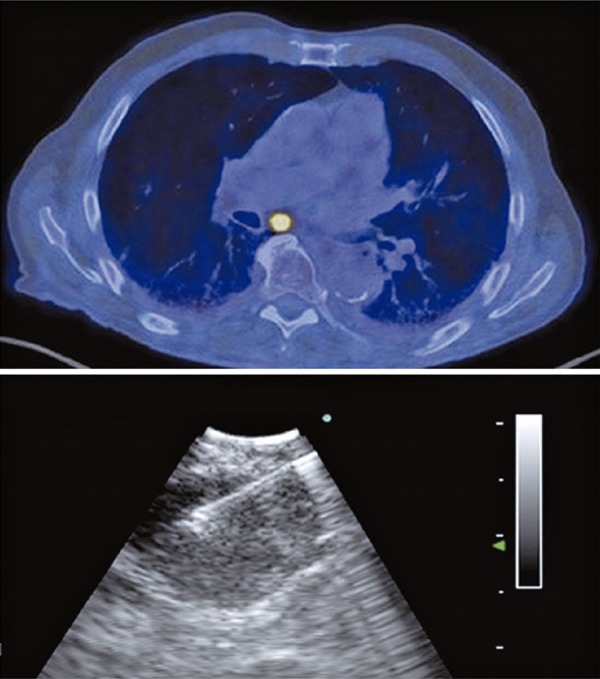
Positron emission tomography (SUV 12.6) and endobronchial ultrasound. Transbronchial needle aspiration with real-time lymph node puncture
